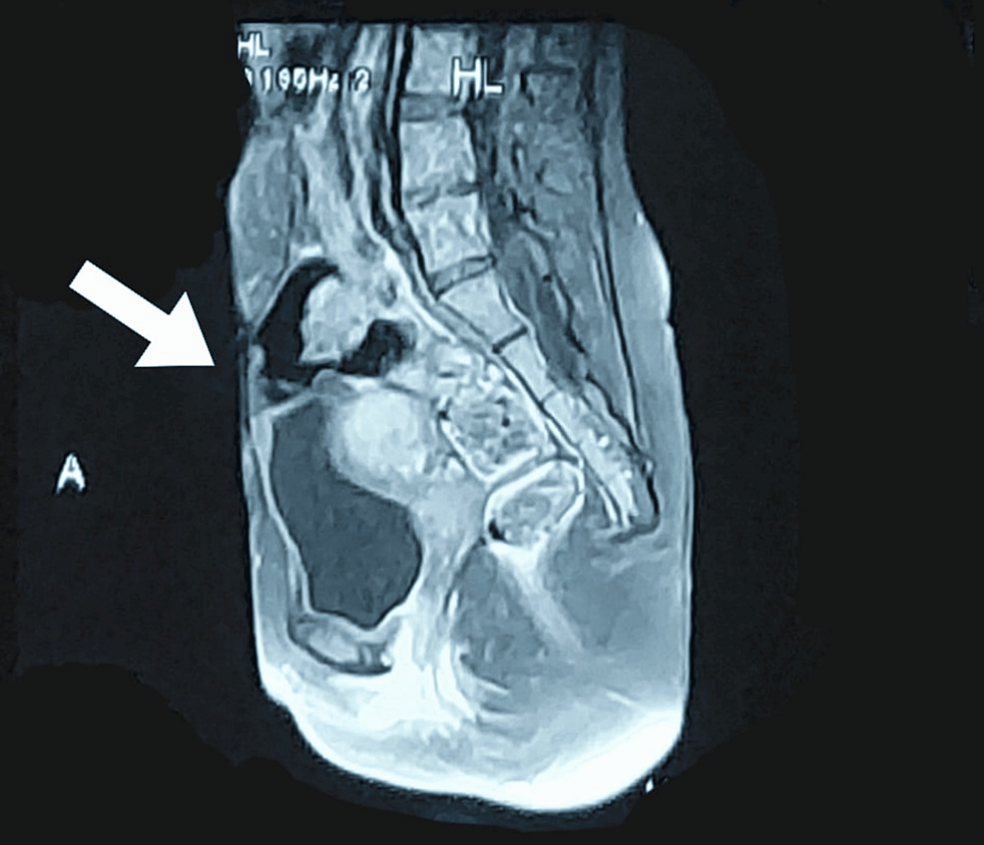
MRI pelvis showing bicornuate uterus, as pointed by the arrow. MRI: magnetic resonance imaging
